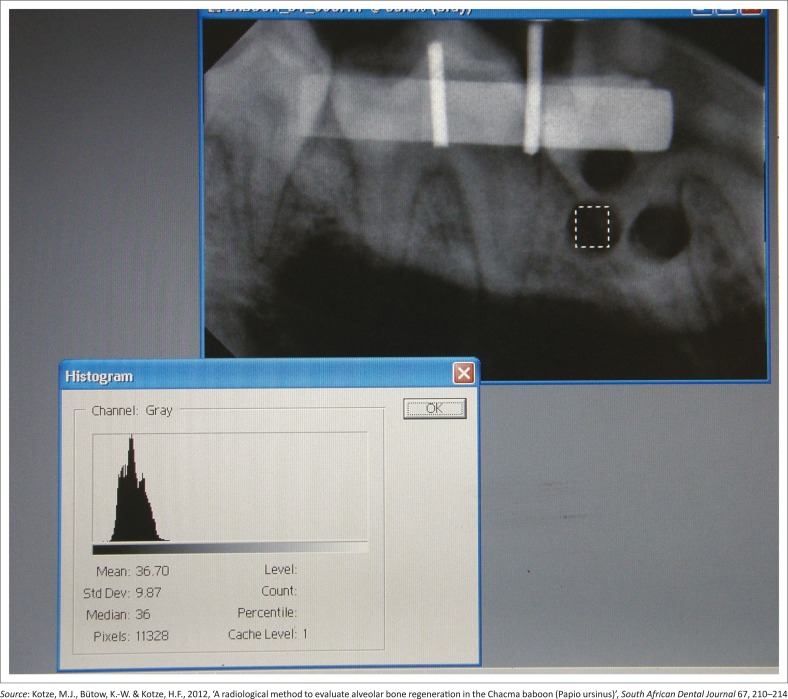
An image of the computer screen displaying the histogram and reflecting the average radiographic intensity of the image of the selected area of the created defect.
